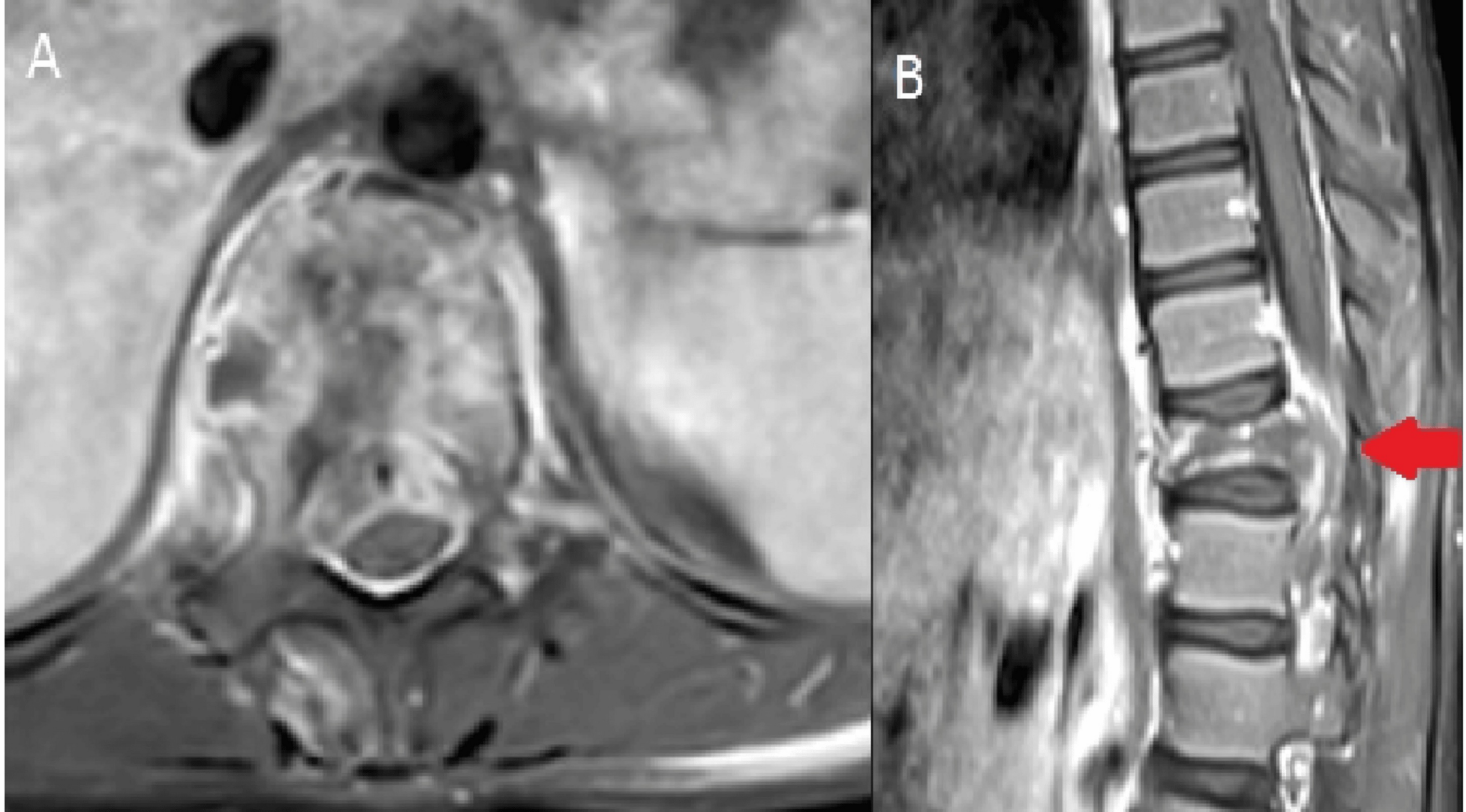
MRI of the thoracic spine showing a T11 vertebral body pathological fracture with an enhancing extraosseous soft-tissue component, causing effacement of the thecal sleeves and cord compression. (A) Axial view. (B) Sagittal view.
